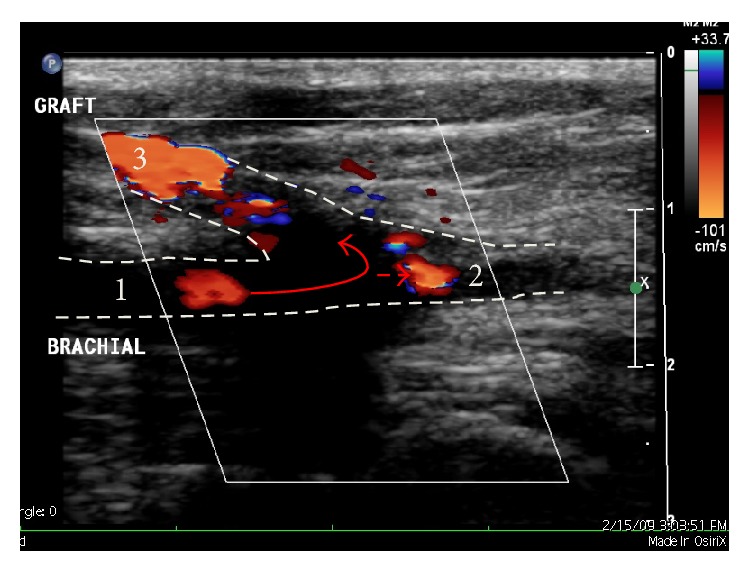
Duplex Doppler ultrasound of the left antecubital fossa demonstrating a significant steal syndrome. Blood enters the proximal brachial artery (1) and >70% is shunted through the PTFE graft (3) with <30% flow through the native distal artery (2).
